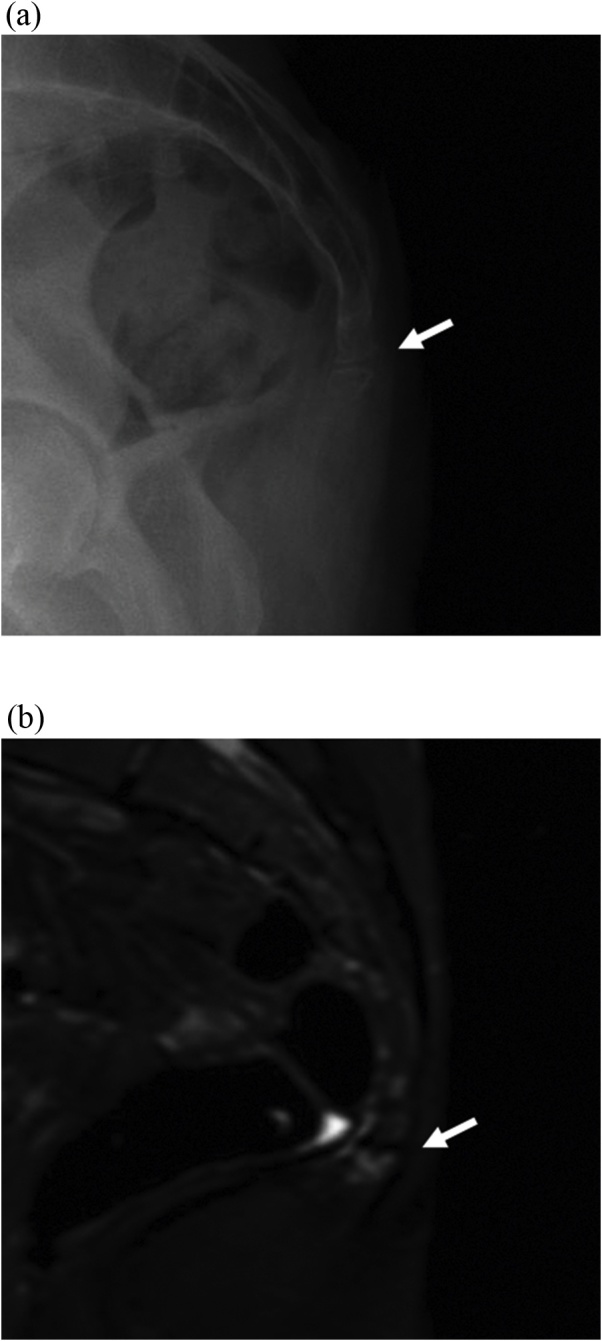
(a) Initial lateral standing coccyx x-ray demonstrating no displacement. White arrow: disruption of coccyx with inline fracture appearing as normal anatomy. (b) MRI coccyx showing marrow edema (white arrow).
